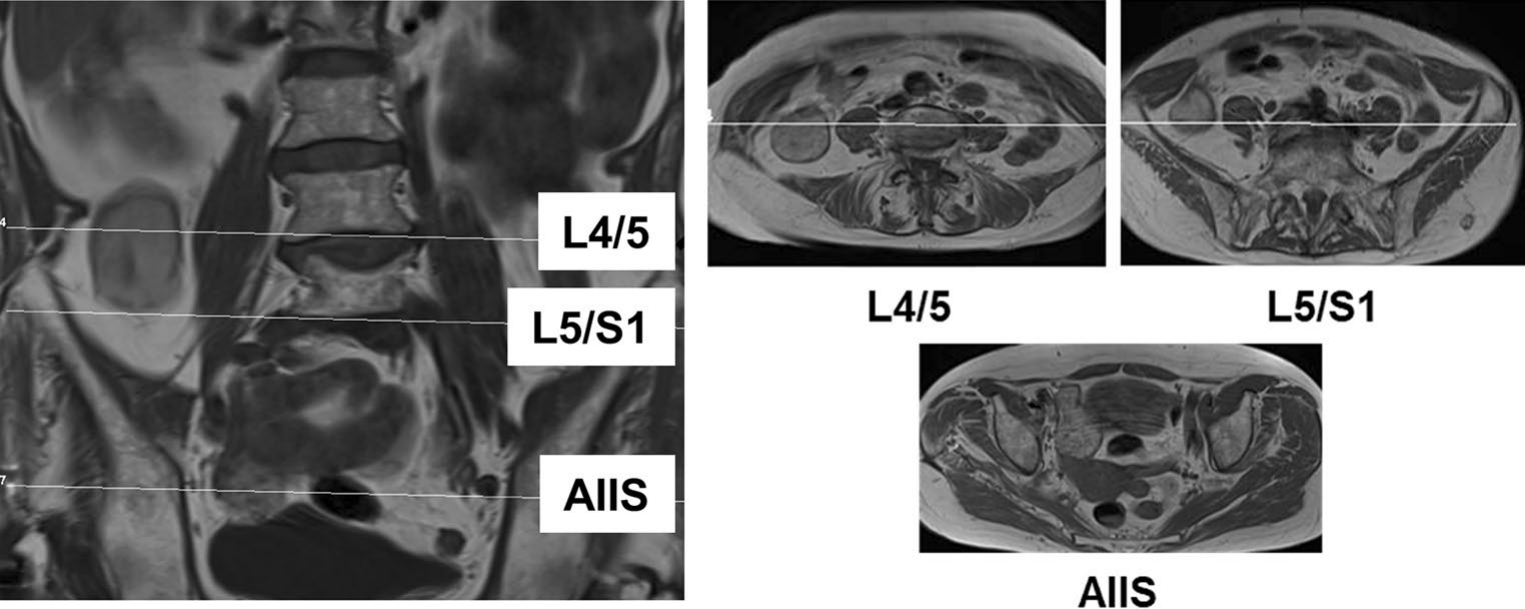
Anatomical landmarks used in MRI-based assessment of fatty infiltration. *MRI* magnetic resonance imaging; *L4/5* lumbar segments 4/5; *L5/S1* lumbosacral segment; *AIIS* anterior inferior iliac spine
